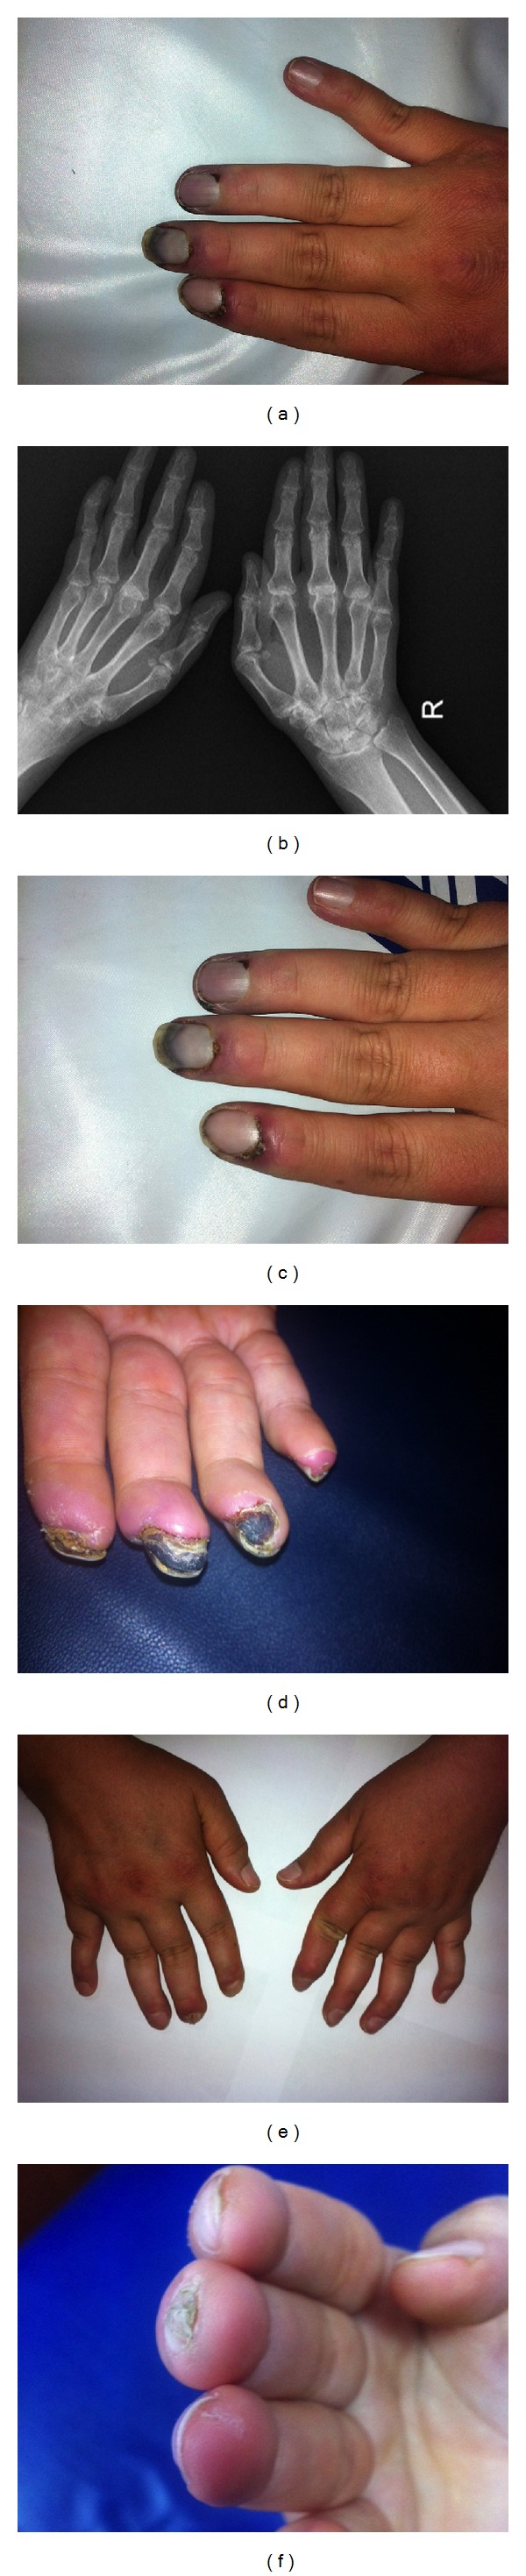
(a) Right hand with swan neck and Z deformity of the thumb (before treatment). (b) Radiograph; AP of bilateral hands suggesting Jaccoud radiological features. (c) Digital ulcers of the second to fourth digits of the right hand (2 weeks after initial treatment with cyclophosphamide). (d) Unresponsiveness to initial treatment demonstrated by a serious progression from ischemic lesions to digital gangrene lesions of the second to fourth digits of the right hand (one month after initial treatment regimen; cyclophosphamide, corticosteroids, and prostaglandins). (e) Response to treatment observed after first cycle of rituximab therapy (5 months following the start of RTX treatment). (f) Complete remission of digital gangrenous lesions after 2 cycles of rituximab therapy (10th month of the RTX treatment protocol (rituximab, glucocorticoid, aspirin, azathioprine, and hydroxychloroquine)).
